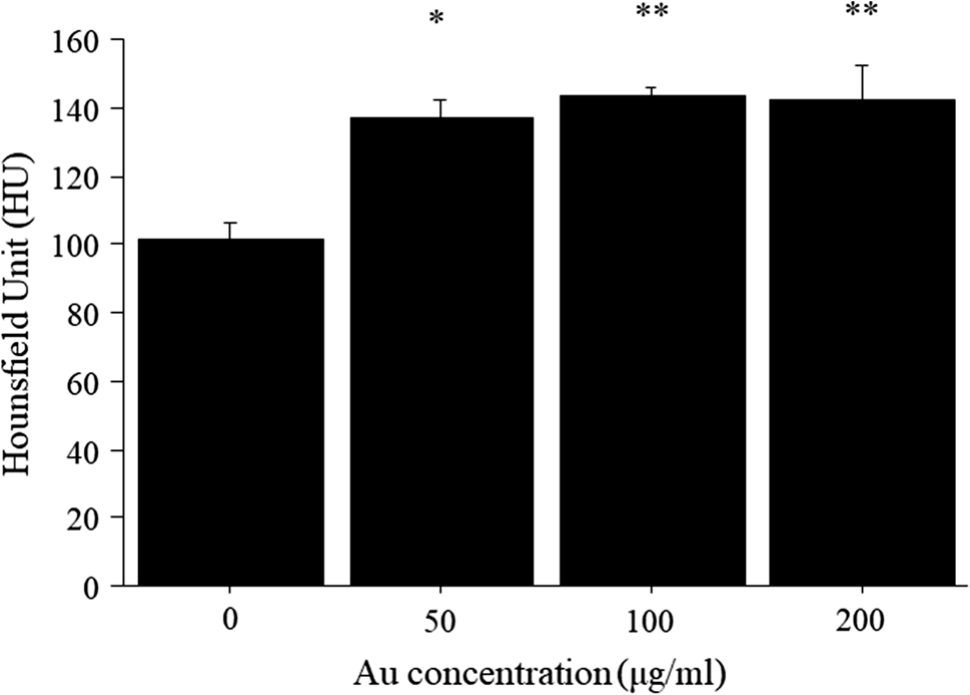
In vitro* CT attenuation values of macrophages incubated with and without GNPs. The CT attenuation values of macrophages incubated with GNPs were significantly higher than those of macrophages without GNPs. p* < 0.03 *vs*. macrophages without GNPs, *p* < 0.04 *vs*. macrophages without GNPs.
